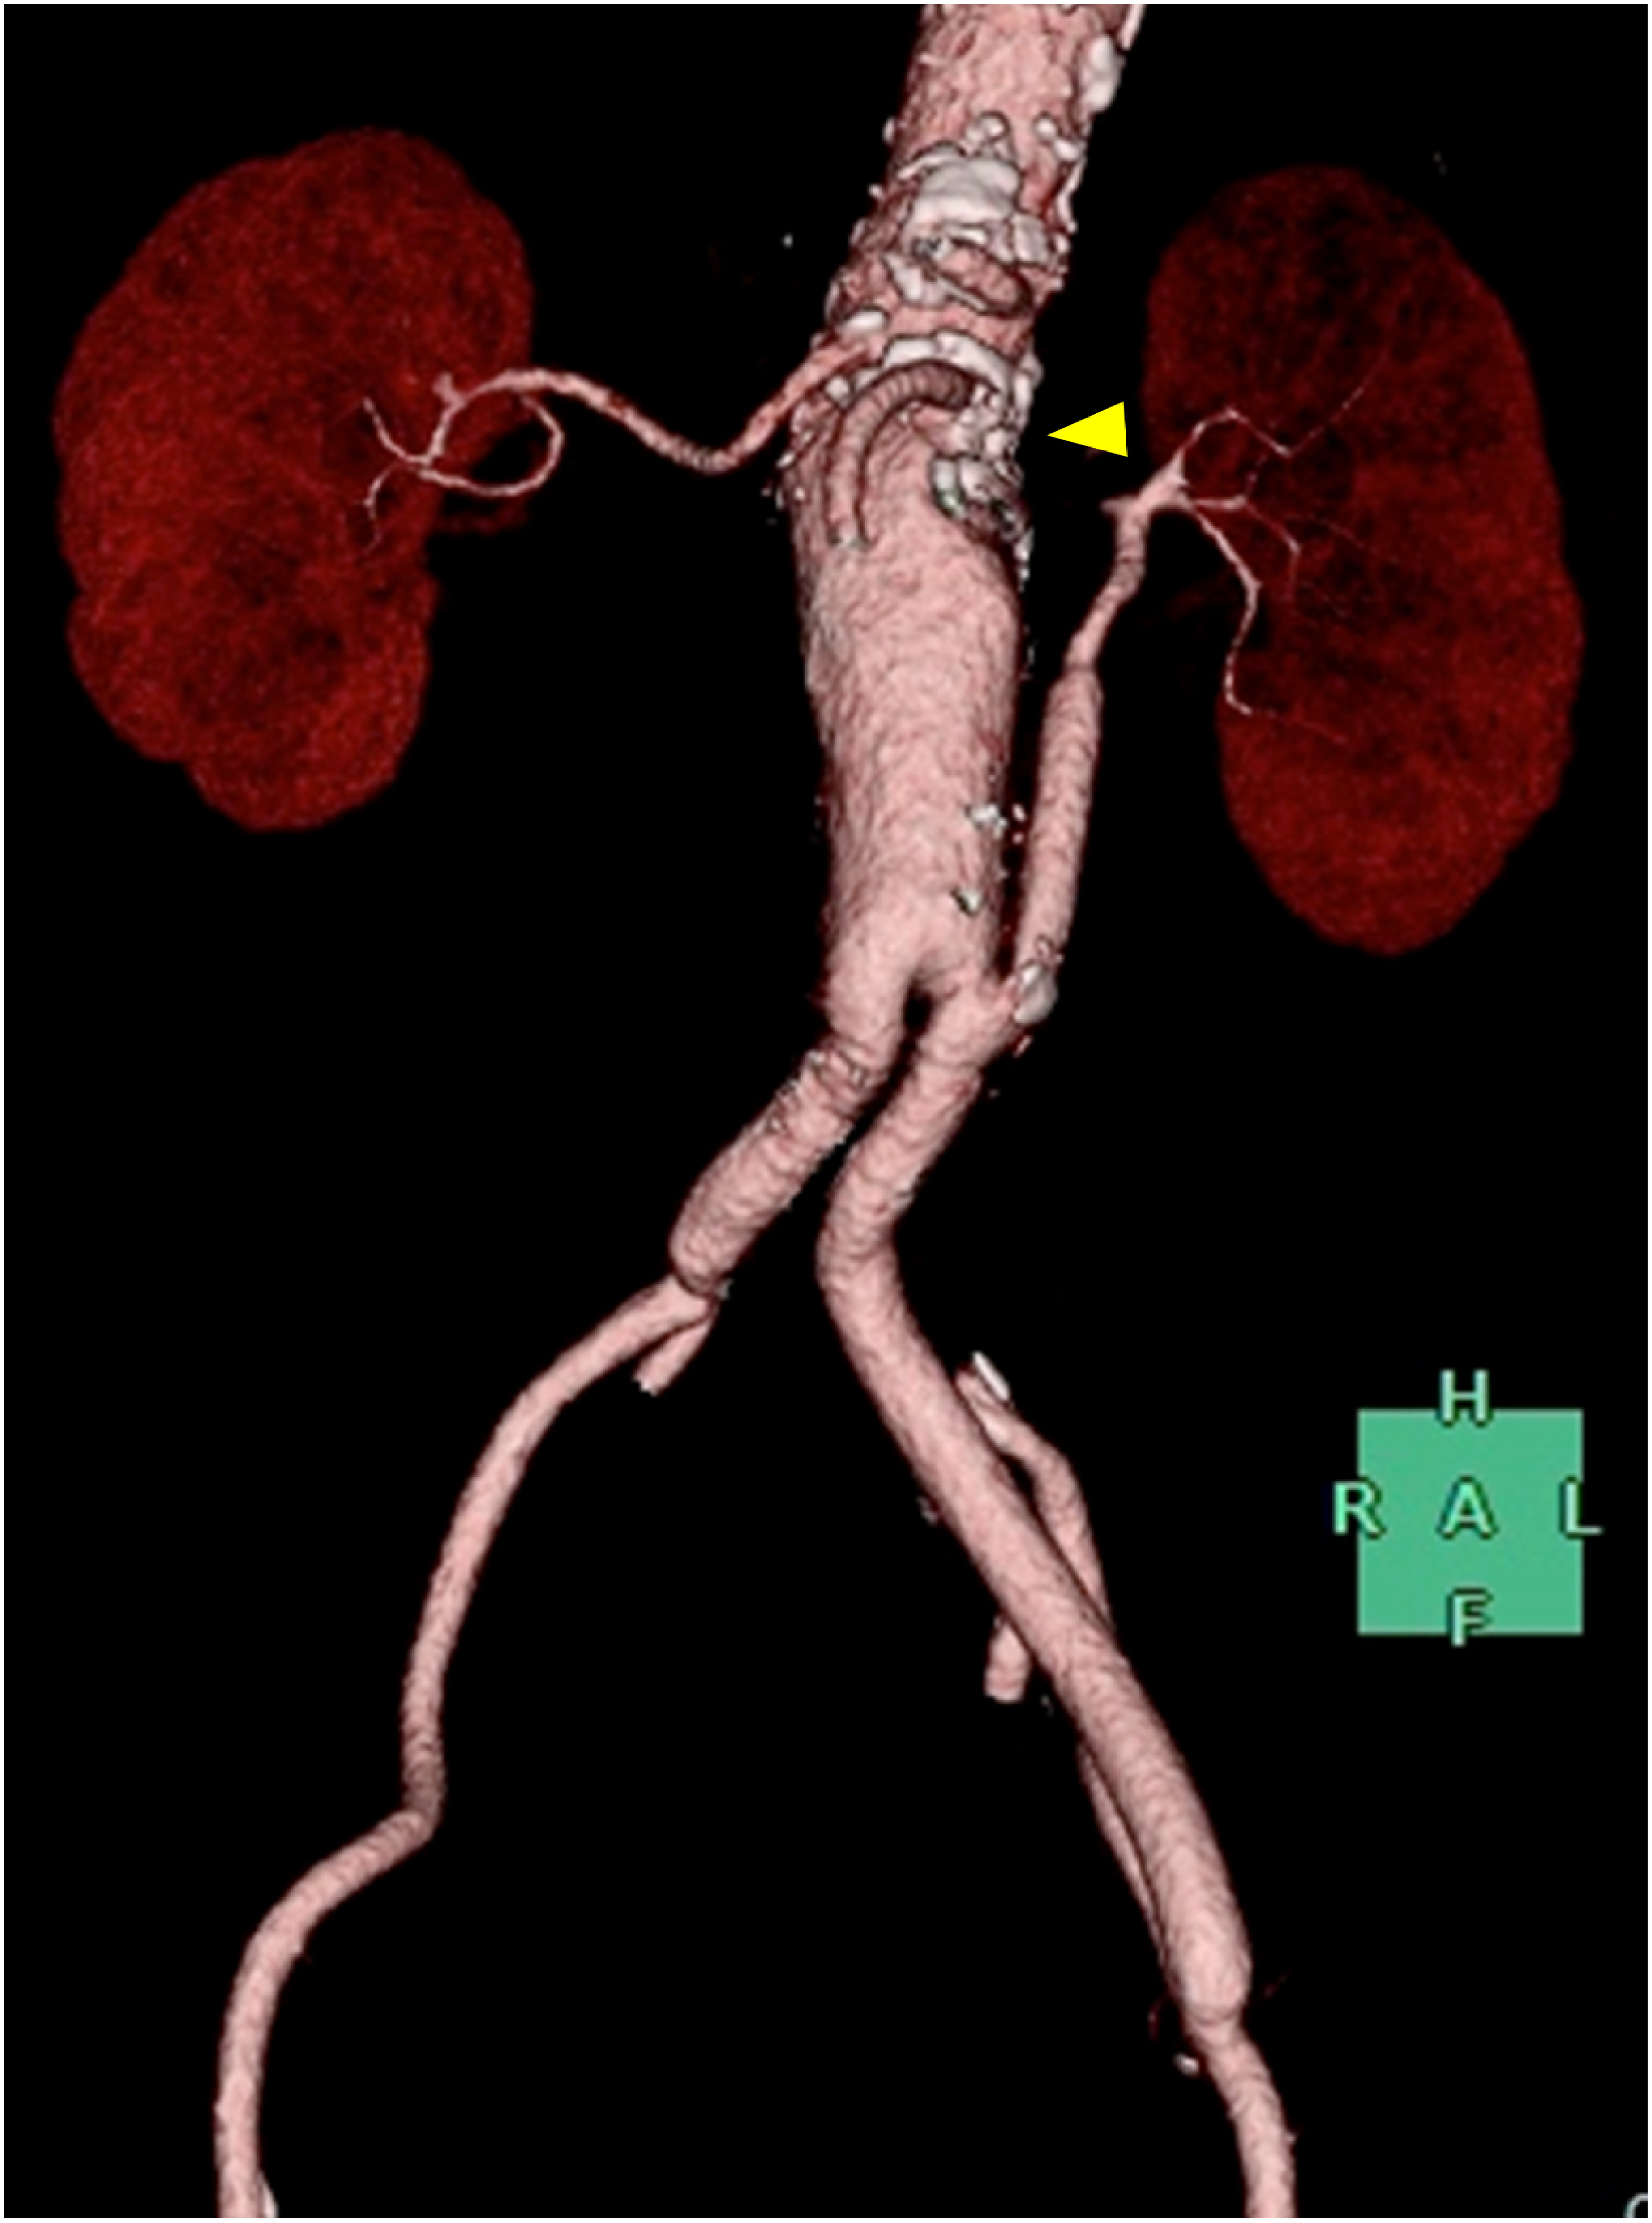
Fig. 3 Postoperative imaging of a tailor-made tapering graft. Contrast-enhanced computed tomography 1 year after surgery. The tailor-made tapering graft was created from Hemashield Gold 18 mm ×9 mm. An arrowhead shows the proximal anastomosis. Note that there is no graft-related complication or thrombus, and both graft limbs were anastomosed to the normal-sized iliac arteries.
